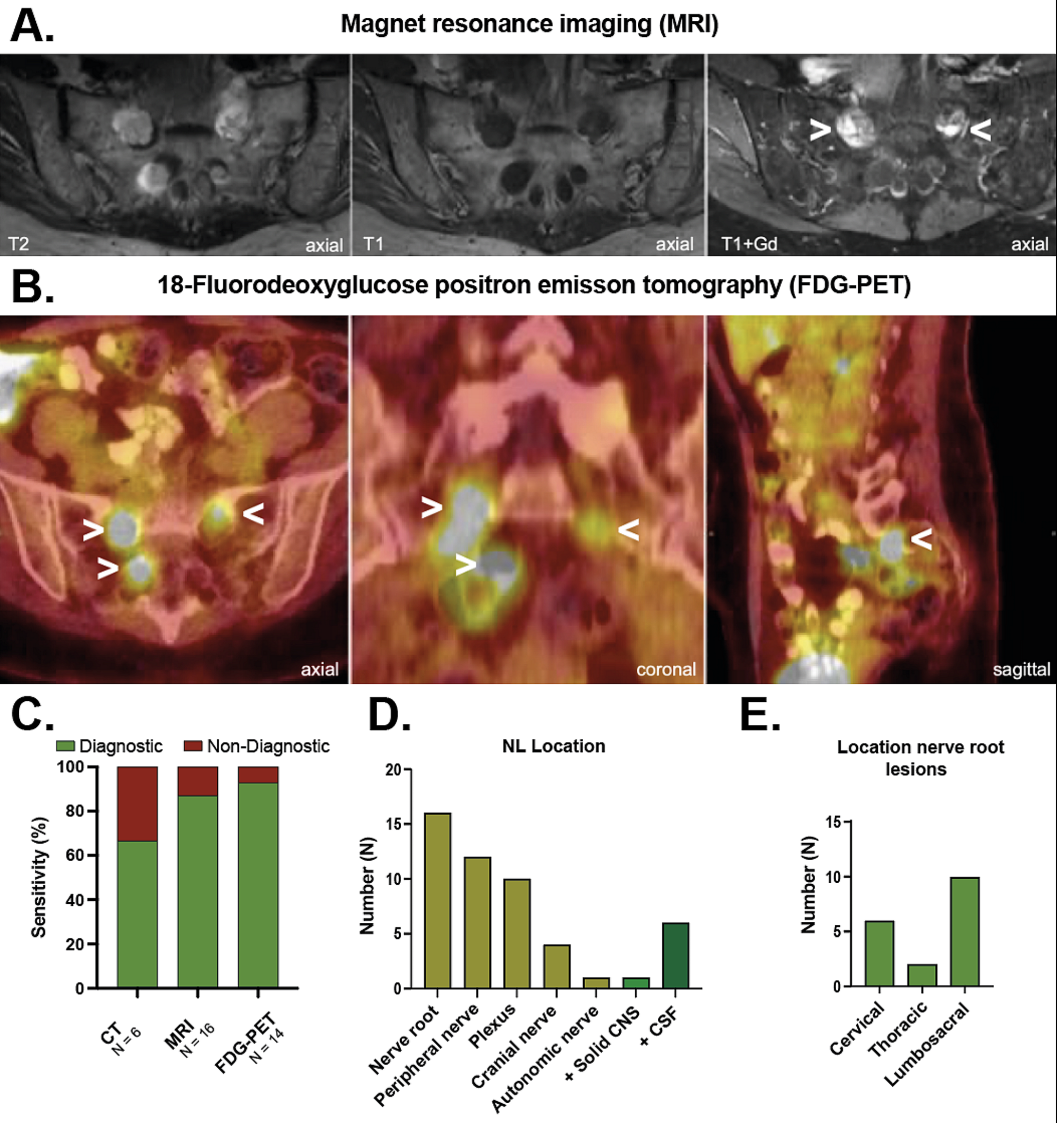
Title: Imaging findings. Legend: MRI (A.) and FDG-PET (B.) imaging findings are depicted in a case of sacral NL. Sequences and planes are indicated. A. Bilateral contrast enhancing S1-nerve root lesions (arrows) were found on MRI. B. Lesions display corresponding FDG-avidity (arrows) on FDG-PET. Of note, this patient had additional congenital cystic CSF-filled enlargement (T2- hyperintensities) of sacral nerve root sleeves (Tarlov cysts) unrelated to NL. C. Sensitivity of various neuroimaging modalities employed in our cohort is shown. FDG-PET exhibited the highest sensitivity (93%). (D) NL location and (E) distribution of nerve root lesions are shown. NHL most frequently infiltrated nerve roots and peripheral nerves
